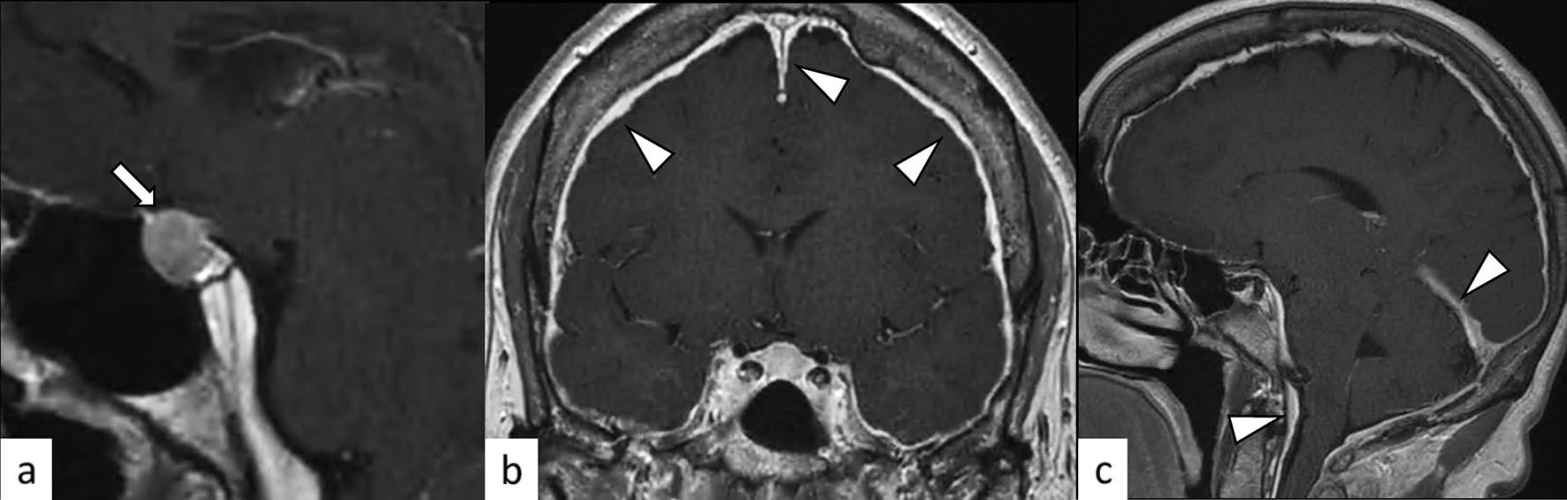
A 62-year-old woman with spontaneous intracranial hypotension. An enlarged pituitary gland is observed on post-contrast T1-weighted sagittal image (a, arrow). Diffuse dural thickening and linear enhancement in both supra- and infratentorial regions are observed (b, c, white arrowheads). A slight downward displacement of the structures appears near the base of the brain, including the hypothalamus, midbrain, pons, and cerebellum
